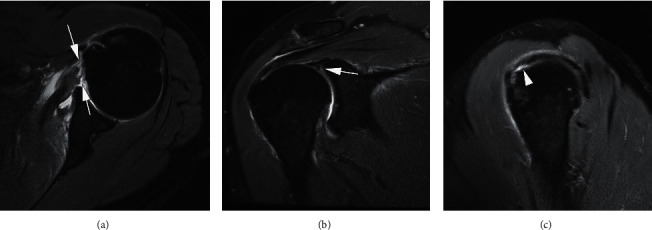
A 46-year-old man with left shoulder pain and limited range of motion after falling during football. Axial T2-weighted FS MRI (a) shows a full-thickness subscapularis tendon tear without relevant retraction from its attachment (arrows). In addition, there is a superior labral tear (arrow) seen on coronal T2-weighted FS MRI (b). Sagittal oblique T2-weighted FS MRI (c) demonstrates a small articular surface tear at the attachment site of the supraspinatus tendon (arrowhead).
